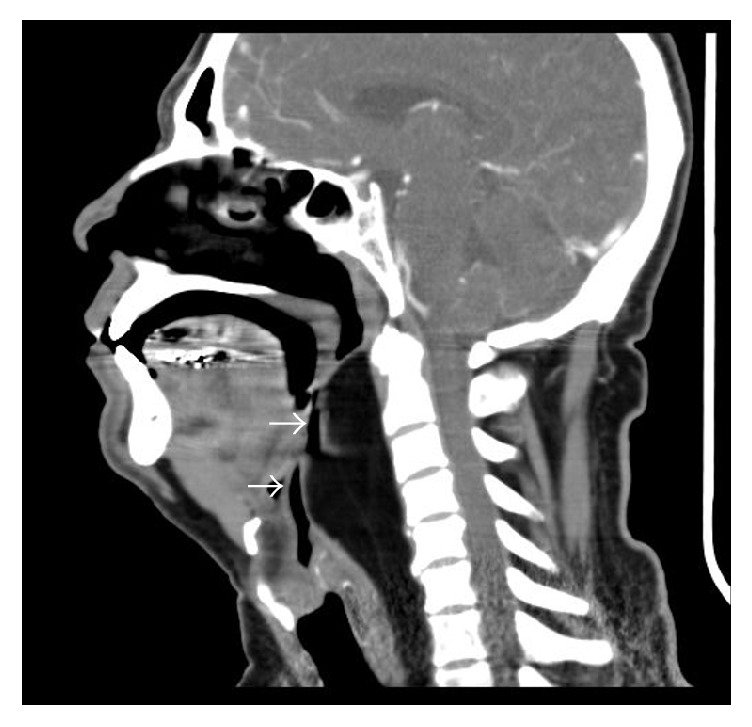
Sagittally reconstructed CT image shows narrowing of the airway (arrows) to a better extent.
